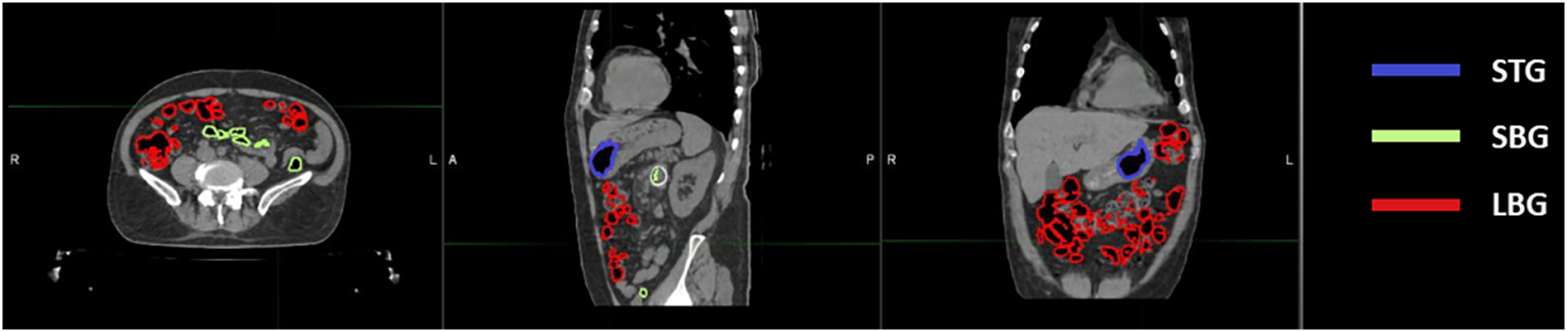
Location of abdominal gas volumes divided by the organ at risk in which they are located. A patient’s CT is shown with gas pockets in the stomach highlighted in blue, those in the small bowel in green and those in the large bowel in red.
